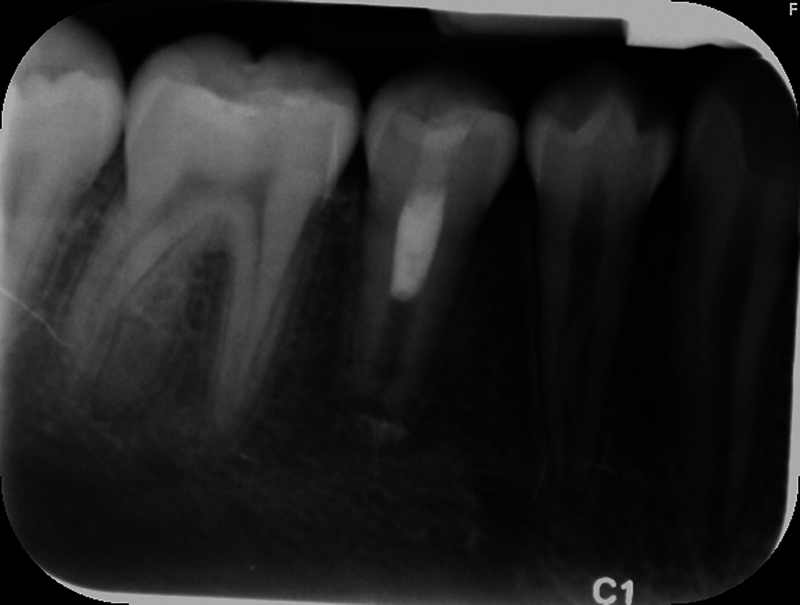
Six-month follow-up periapical radiograph.
